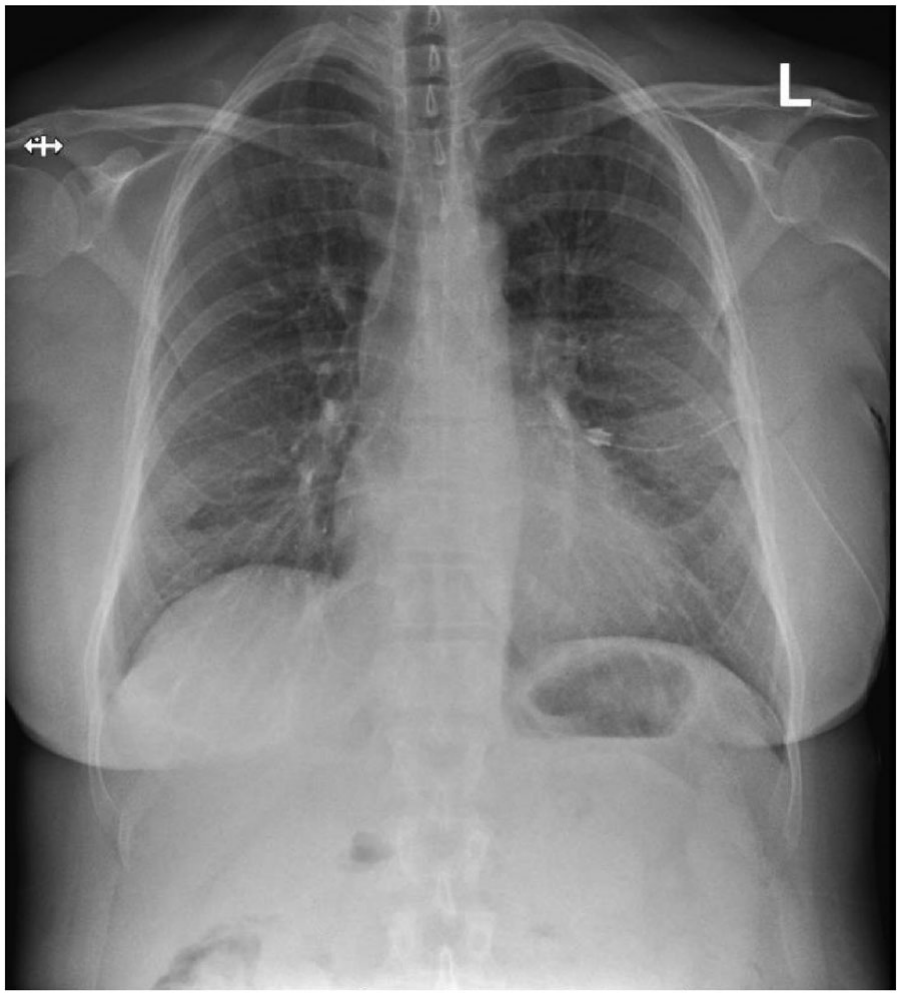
Chest X-ray after insertion of a chest drainage tube showing resolution of the pneumothorax.
